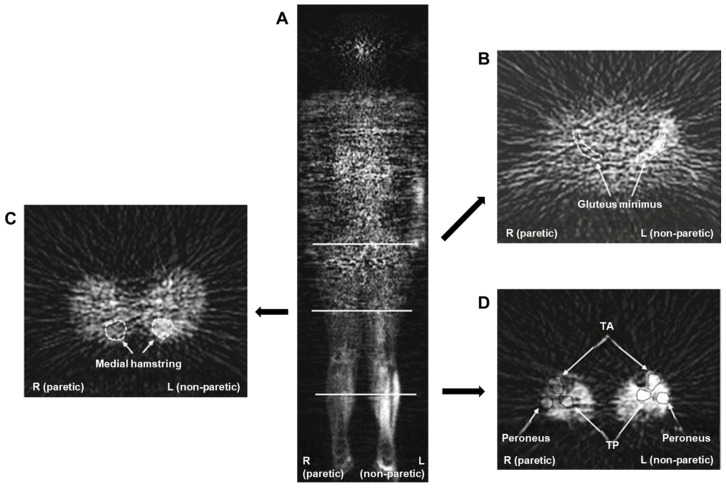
Representative images of whole-body positron emission tomography (PET) from a hemiparetic participant. (A) The coronal PET image (middle) is captured 6 cm in front of the subject’s posterior, depicting minimal [^18^F]fluoro-2-deoxy-D-glucose ([^18^F]FDG) uptake in the muscles of the affected (right) lower limb and elevated [^18^F]FDG uptake in the muscles of the unaffected (left) lower limb. This image provides an anterior–posterior view. (B) PET image in the axial plane of the pelvis showing reduced [^18^F]FDG uptake in the gluteus minimus muscle of the affected (right) lower limb. This image provides an inferior–superior view. (C) Axial PET image of the thigh showing increased [^18^F]FDG uptake in the medial hamstring muscle of the unaffected (left) lower limb. (D) Axial PET image of the lower leg showing reduced [^18^F]FDG uptake in the muscles of the affected (right) lower limb. TA, tibialis anterior muscle; TP, tibialis posterior muscle. Reproduced from [31], copyright © 2015, The Tohoku Journal of Experimental Medicine.
